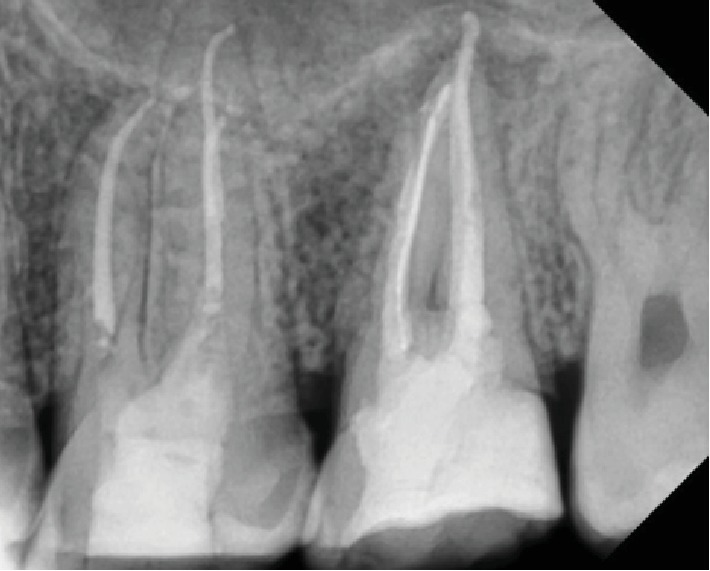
Periapical X-ray after 2 weeks of obturation.
